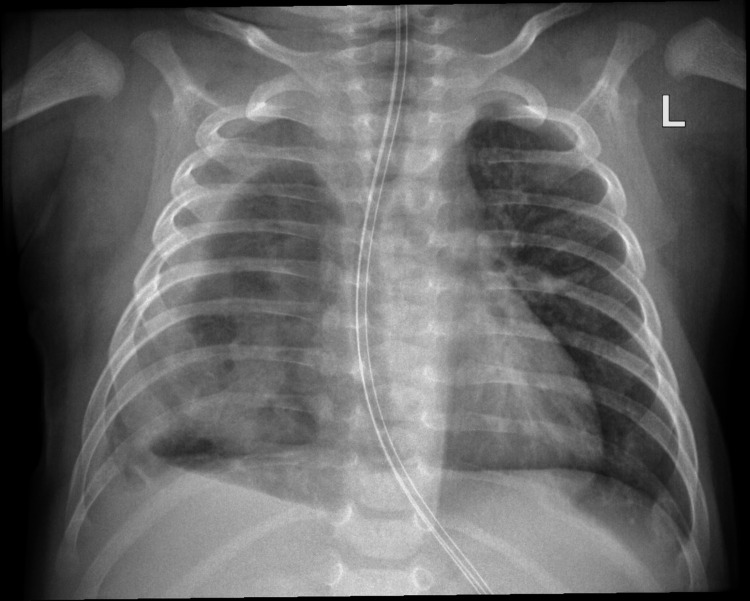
A chest X-ray taken a week after surgery showed fluid levels in the right pleural cavity along with pneumothorax and atelectasis of the right lung.
